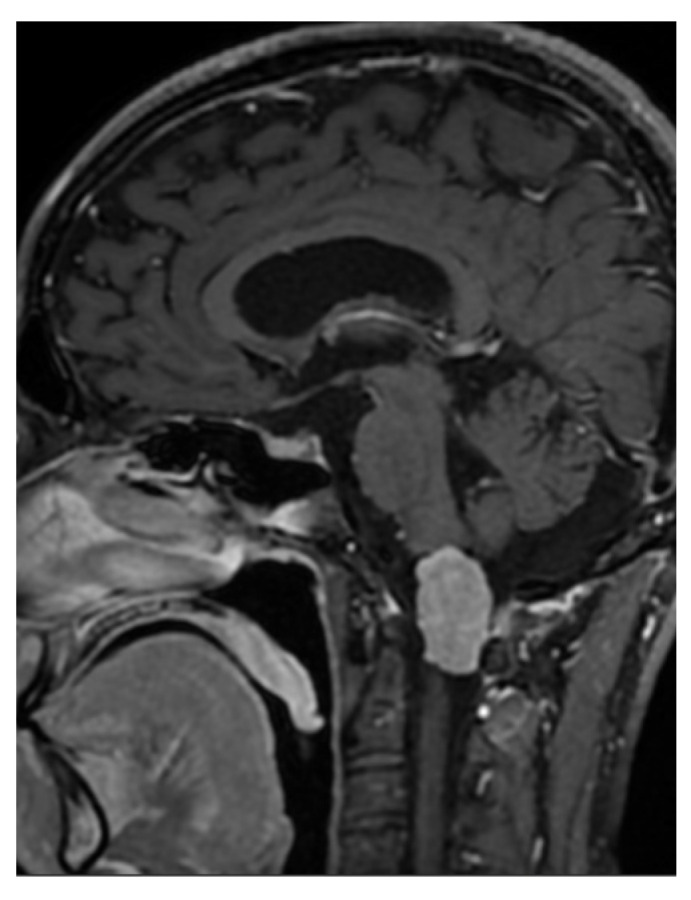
Single sagittal scan of a right-sided foramen magnum meningioma with mild brainstem modeling, MRI T1 + C.
